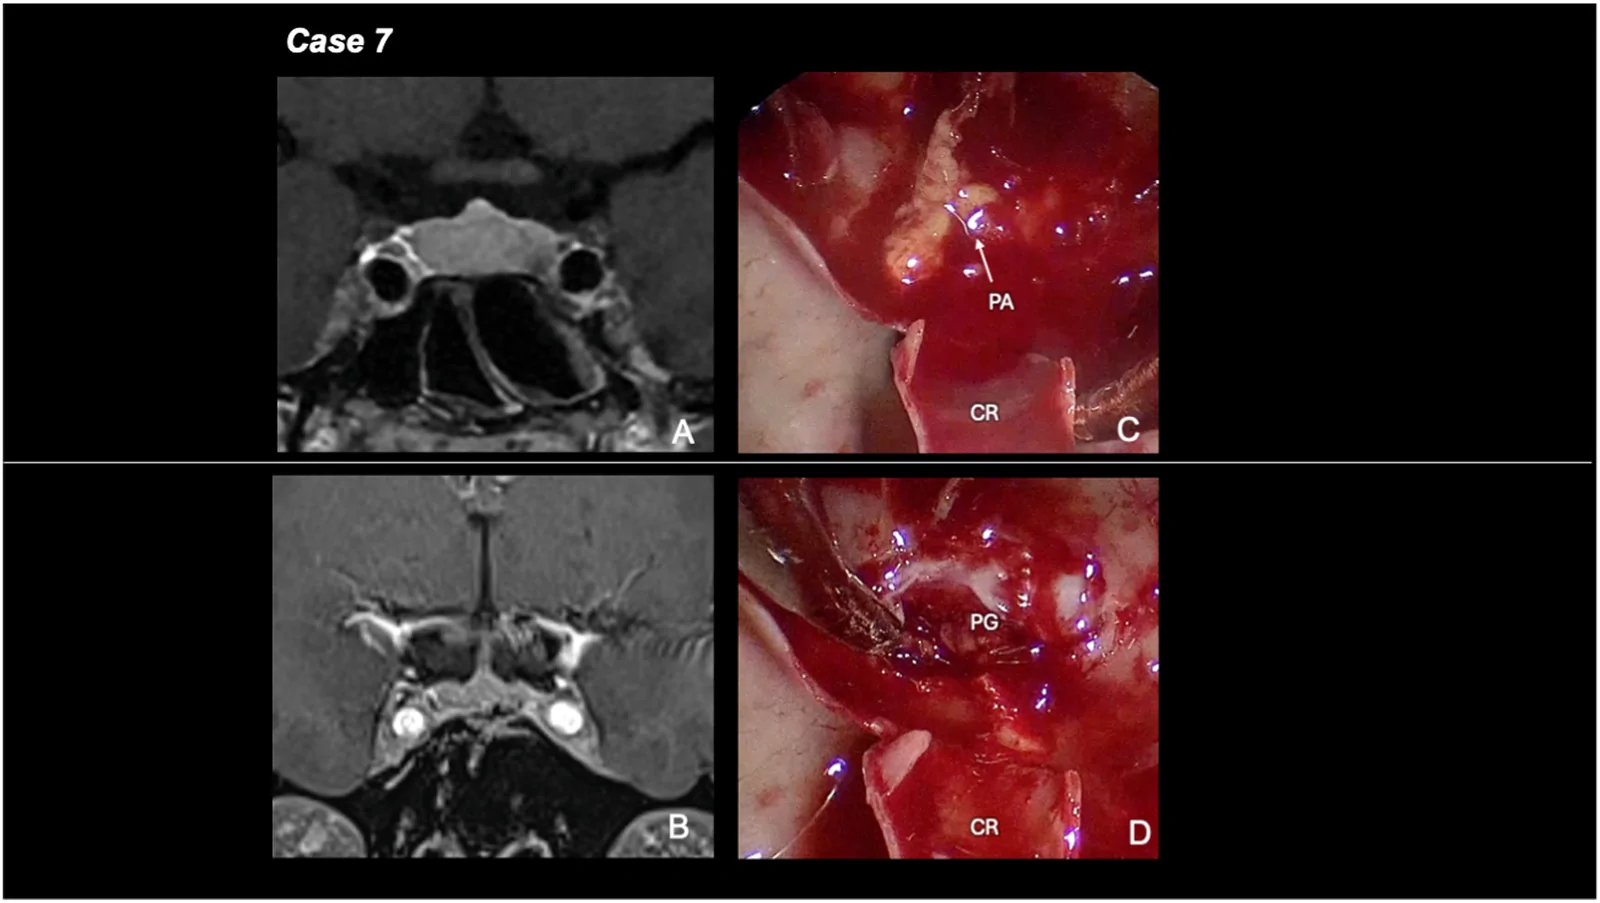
MRI-negative with intraoperative adenoma identification (Case 7) (A) Preoperative coronal T1-weighted MRI showing no identifiable pituitary lesion. (B) Postoperative coronal MRI at 4 months showing no residual. (C) Intraoperative image demonstrating identification of a small right-sided adenoma during pituitary exploration. (D) Intraoperative view of the surgical cavity following enlarged adenomectomy. *Abbreviations*: CR, clival recess; PA, pituitary adenoma; PG, pituitary gland.
